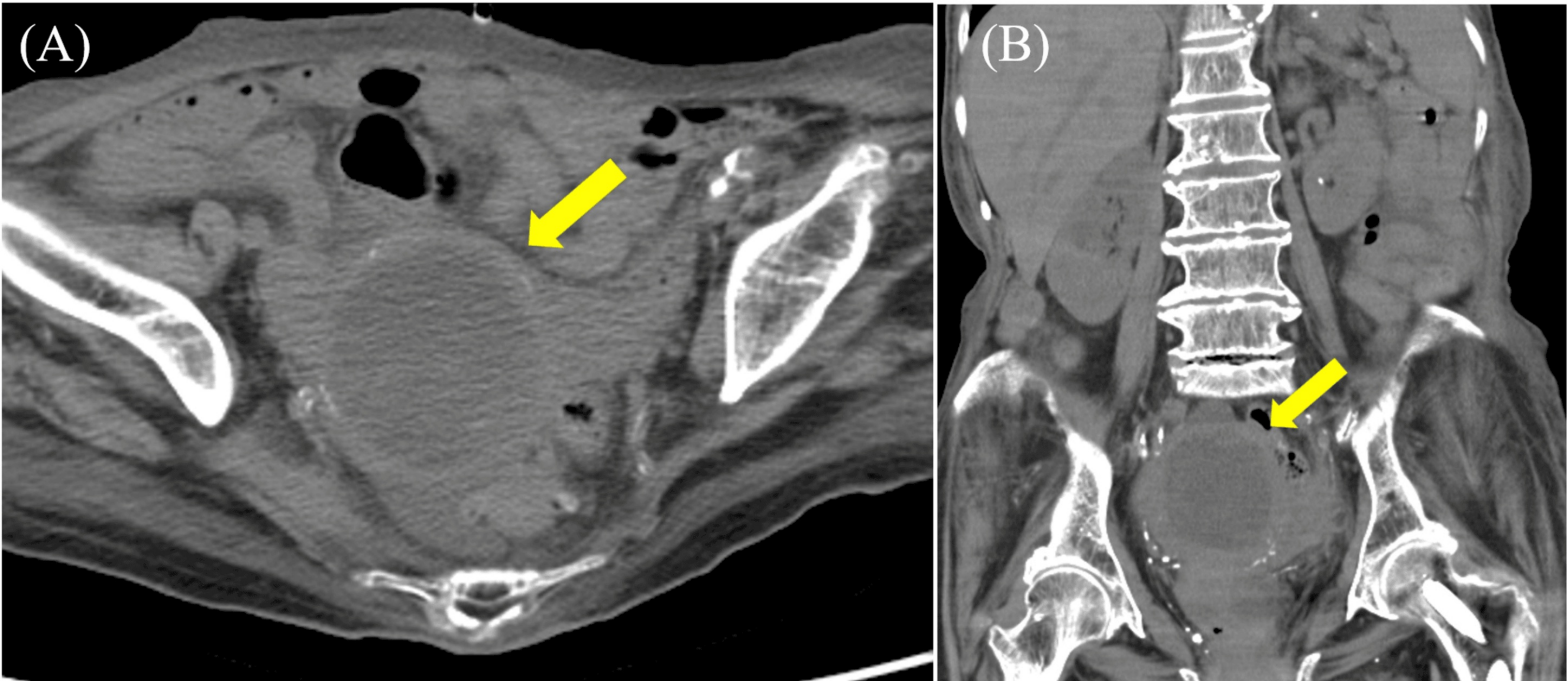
Pelvic computed tomography (CT) findings. Pelvic CT demonstrating a distended uterine cavity filled with fluid or purulent material, appearing as a well-defined, fluid-density structure within the uterus. (A) Axial section; (B) coronal section.
